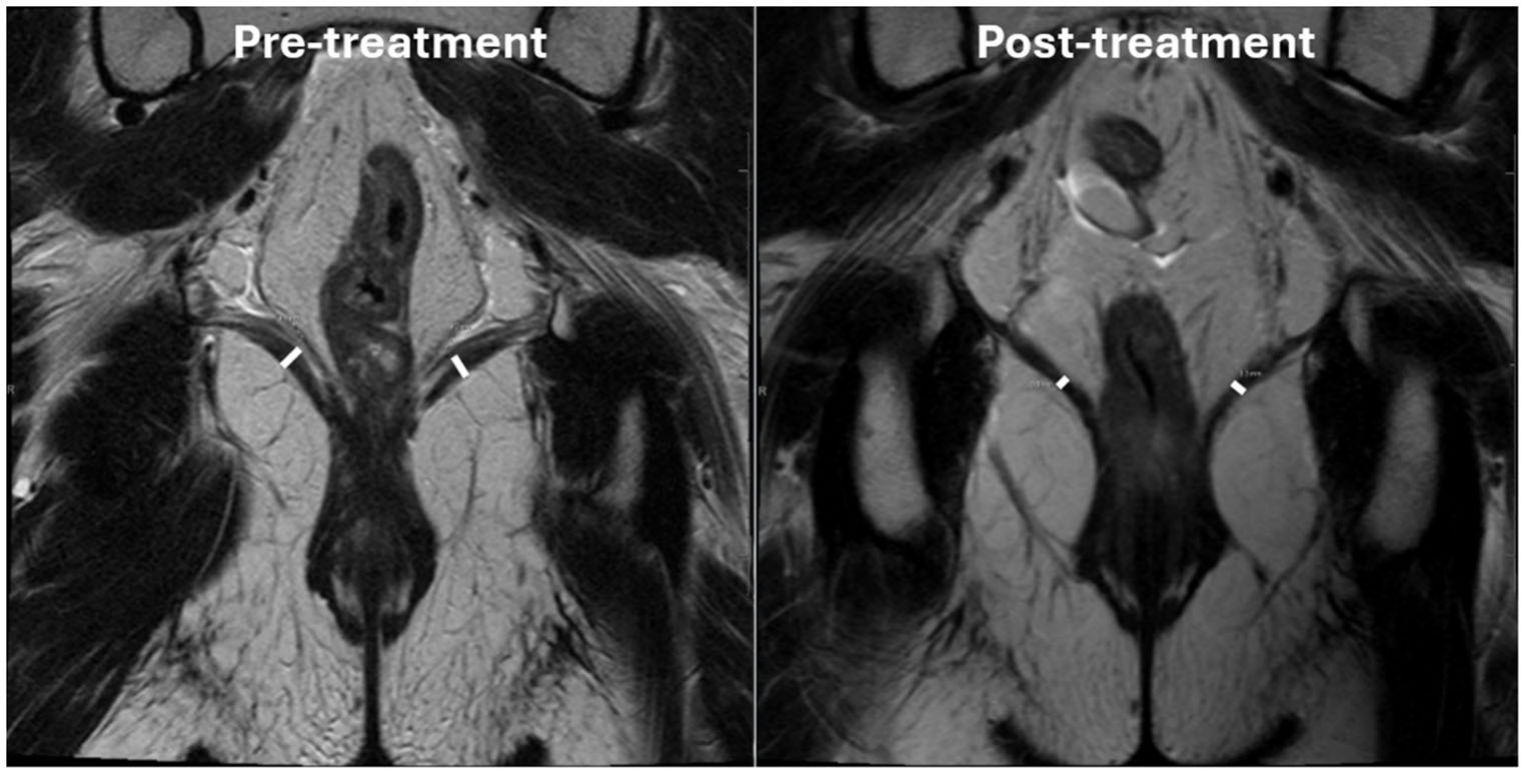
Pre-treatment and 12-month post-radiation MRI of a representative patient.
